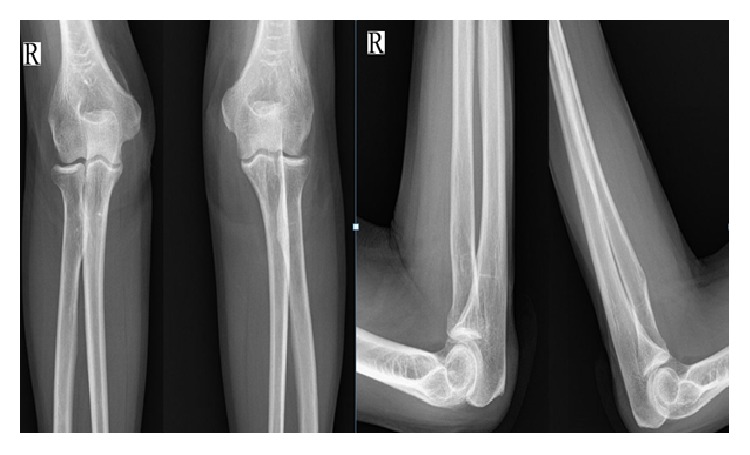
Images of X-ray, right and left elbow: The direct preoperation radiographies showed no significant changes or calcification (9/5/16).
